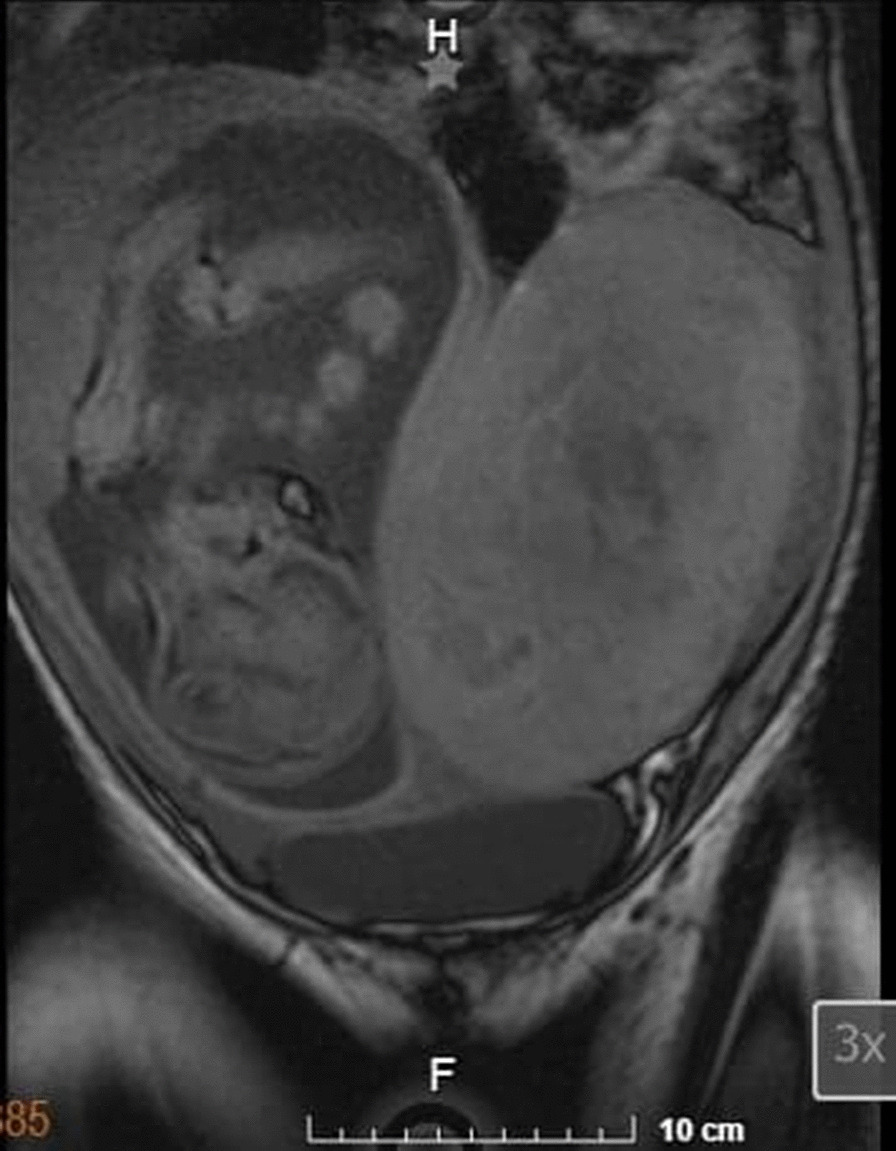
Abdominal-pelvic magnetic resonance imaging, T1- and T2-weighted sequences. Mass effect findings. Image shows left adnexal mass in extensive contact with the uterine corpus that conditions right confinement of the uterus, eccentric displacement of the small intestine, mild compression of the bladder without infiltration, and left ureteral compression
